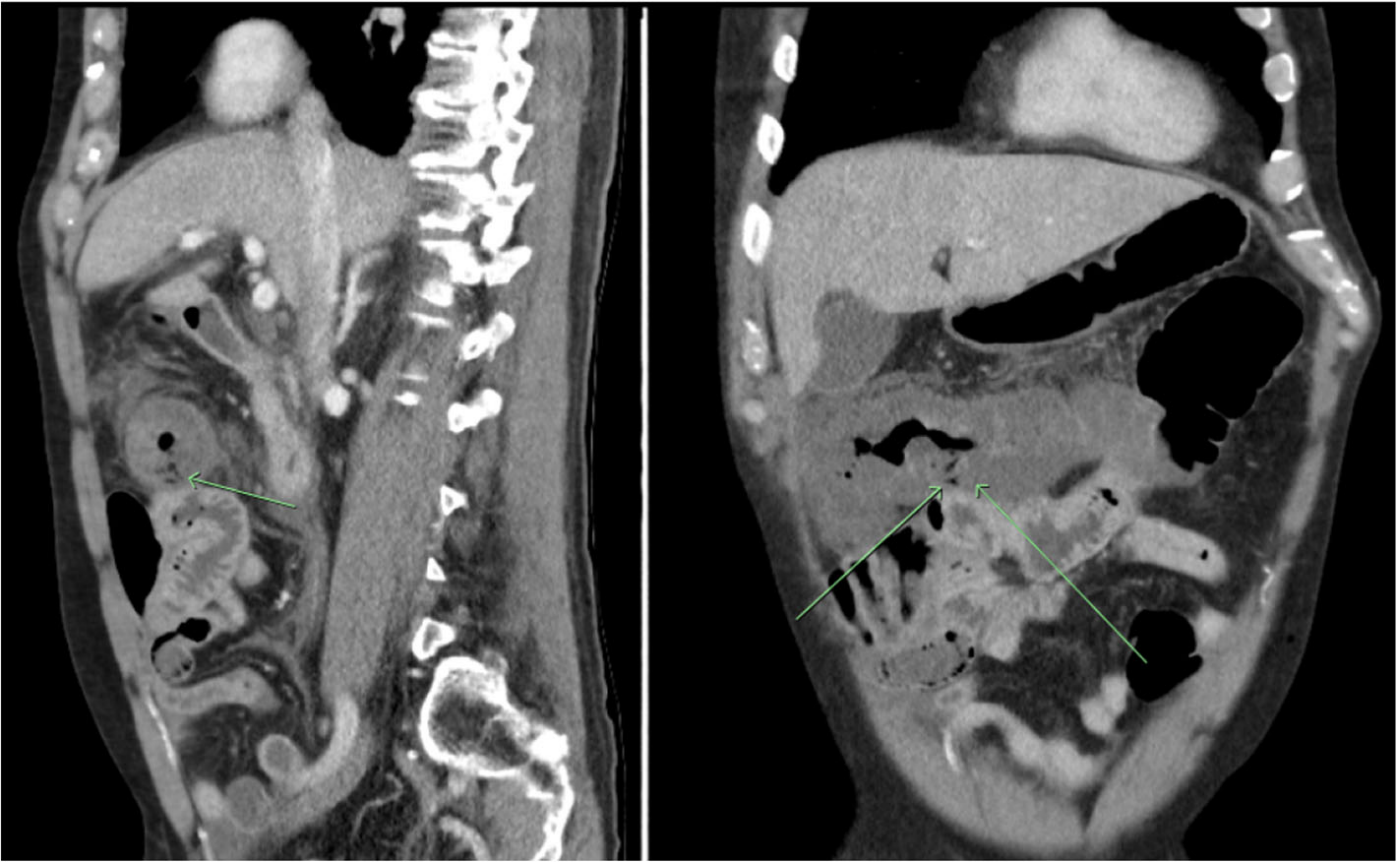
Abdominal CT, sagittal and coronal views, showing mural thickening of the proximal transverse colon and hepatic flexure with arrows highlighting visible intramural gas
